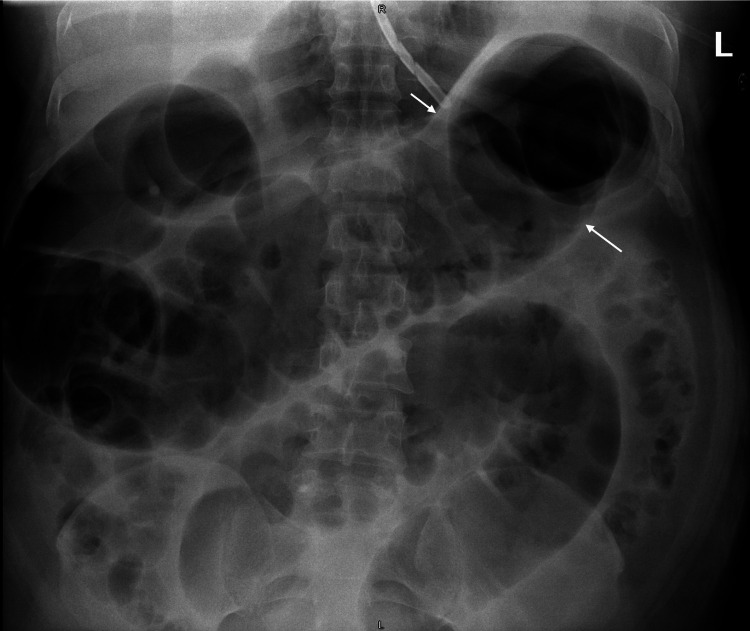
Abdominal x-ray shows colonic dilatation (White arrows)
